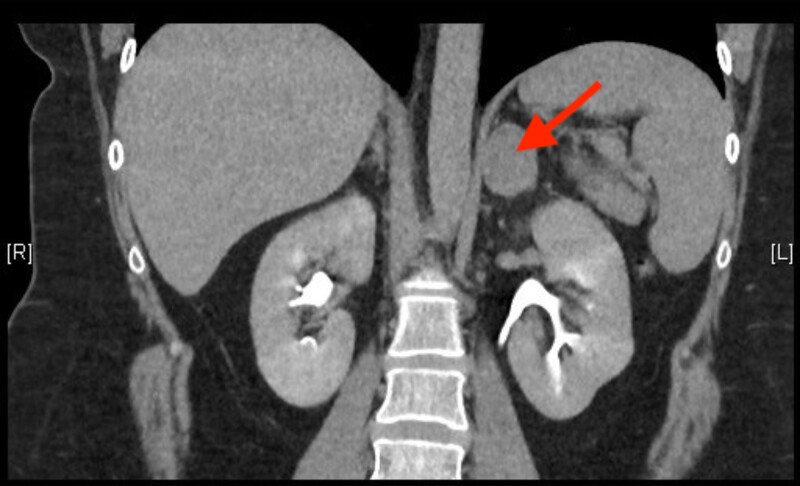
Coronal view of adrenal computed tomography scan demonstrating left adrenal lesion (red arrow).
